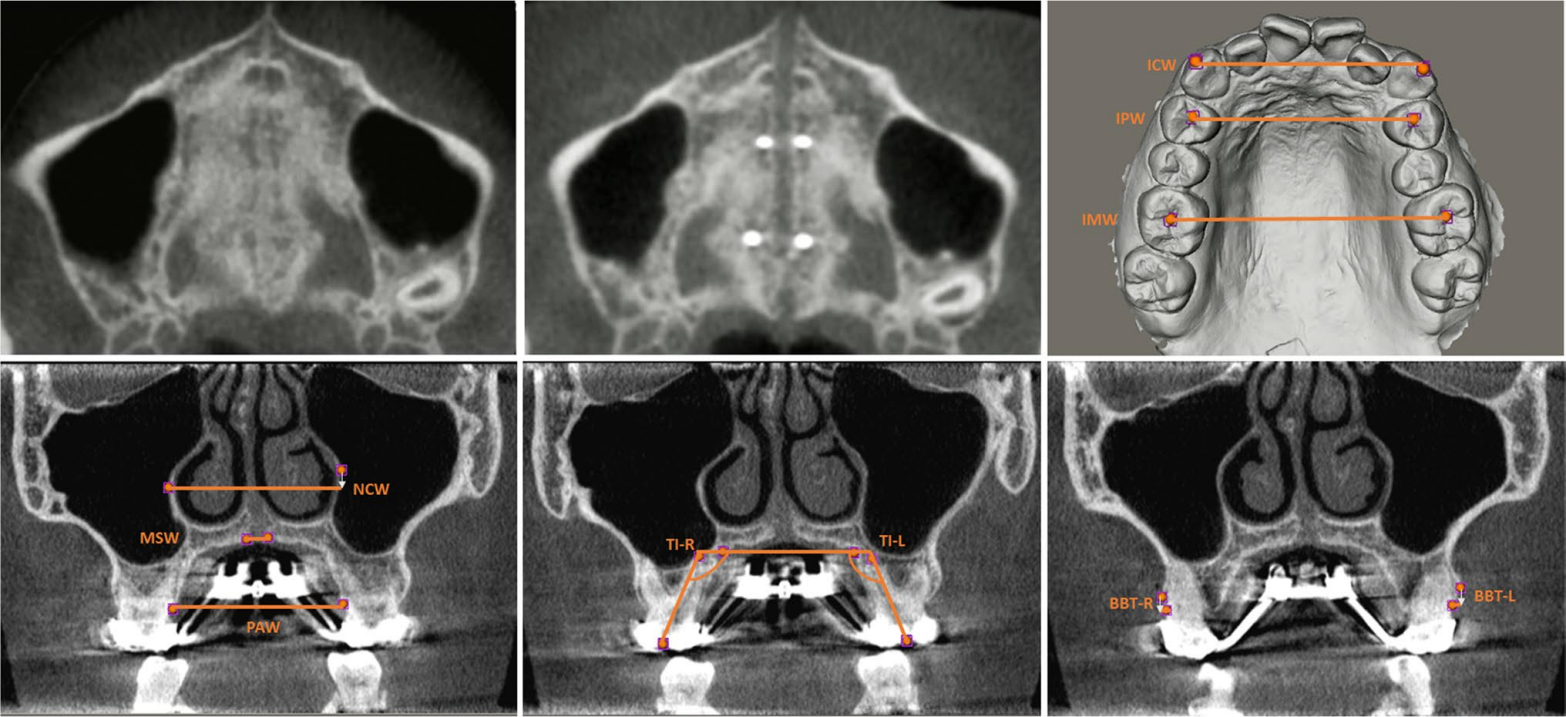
The hard palate at T0 and T1, and skeletal and dentoalveolar measurements on CBCT and IOS. (A: *upper left*) Hard palate at T0, before midpalatal suture separation, on an axial CBCT slice. (B: *upper middle*) Hard palate at T1, with separated midpalatal suture, on an axial CBCT slice. (C: *upper right*) Measurement of IMW, IPW, and ICW on an intra-oral scan. (D: *lower left*) Measurement of MSW, PAW, and NCW on a coronal cross-sectional CBCT slice through the buccal groove of the M1. (E: *lower middle*) Measurement of TI left and right on a coronal cross-sectional CBCT slice through the buccal groove of the M1. (F: *lower right*) Measurement of BBT left and right on a coronal cross-sectional CBCT slice through widest portion of the mesiobuccal root of the M1
